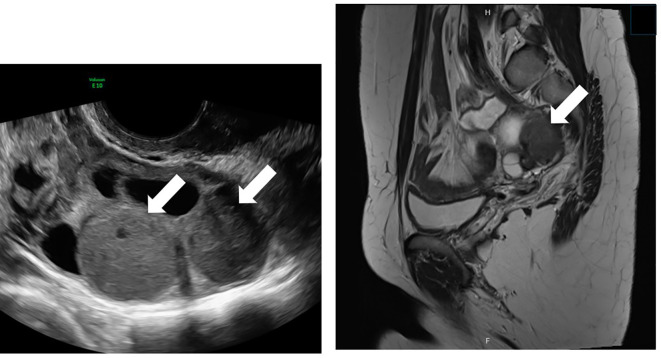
Left: Transvaginal ultrasound image of right ovary with two endometriomas. Right: Sagittal view of T2-weighted MR image of the same ovary with T2-hypointense lesion representing endometrioma.
